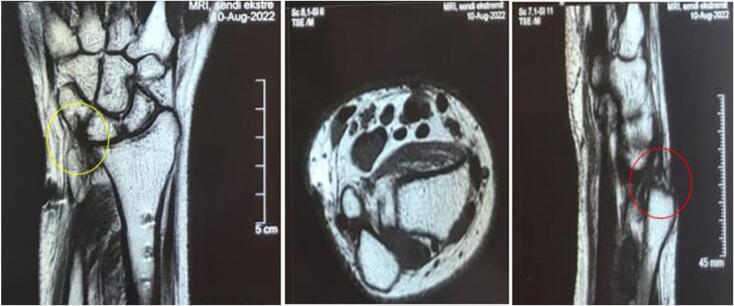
Initial right forearm X-ray examination after implant removal when DRUJ being symptomatic.
